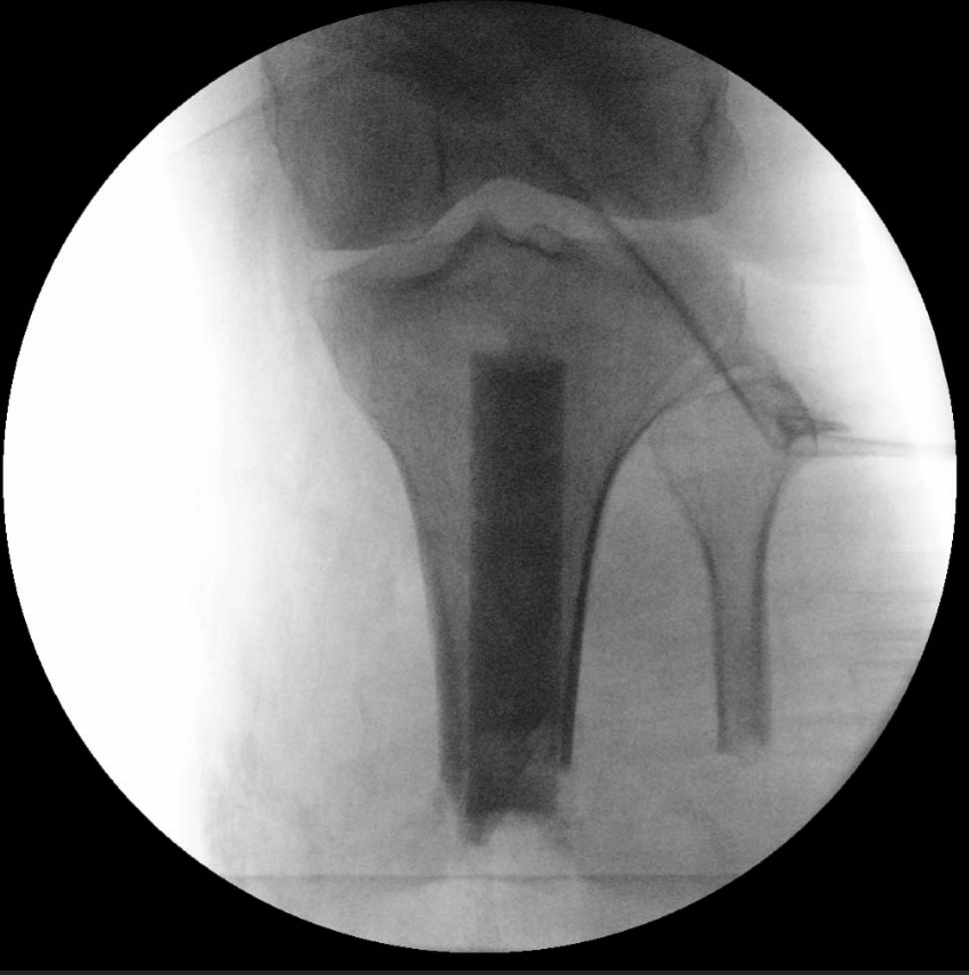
An intraoperative AP radiograph of the left knee showing placement of the antibiotic dowel in the intramedullary canal of the tibia.
